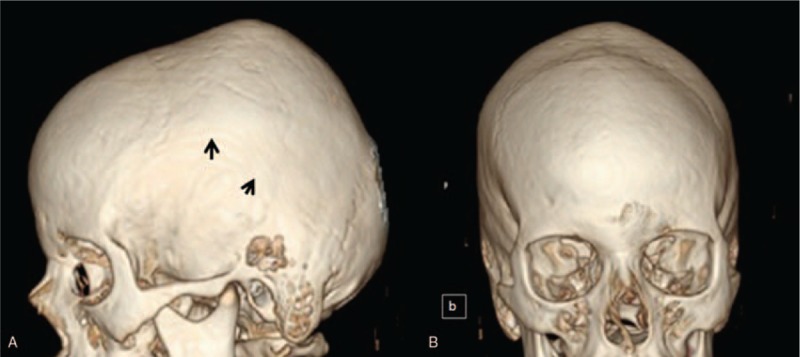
(A) 3D reconstruction CT of the proband skull showed severe expansion of the skull posterior to the coronal sutures because of mixed synostosis of the squamosal sutures (arrows) and the anterior part of the sagittal suture. The overall skull phenotype is somehow brachycephalic. (B) 3D reconstruction CT of the proband anterior view showed the massive bulging over the synostosed sagittal suture. CT = computed tomography.
